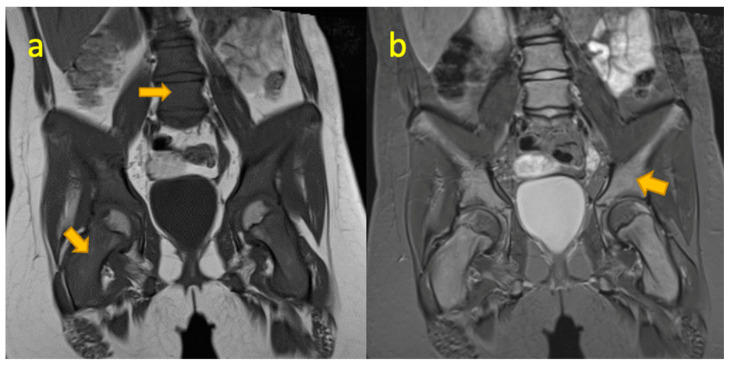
(a,b) Coronal T1W and STIR MRI images of the pelvis in a nine-year-old girl demonstrate diffuse bone marrow signal abnormality consistent with marrow infiltrative process, later proved to be acute B-cell lymphoblastic leukaemia.
